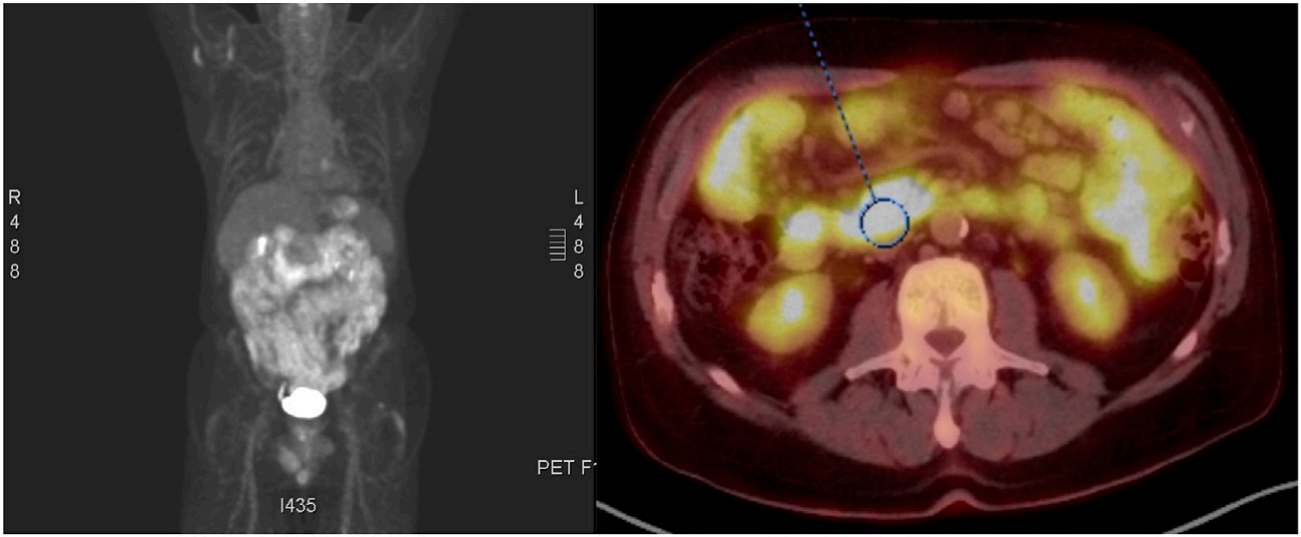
PET-CT demonstrating hypermetabolic , diffuse wall thickening of the stomach and throughout the small bowel.
